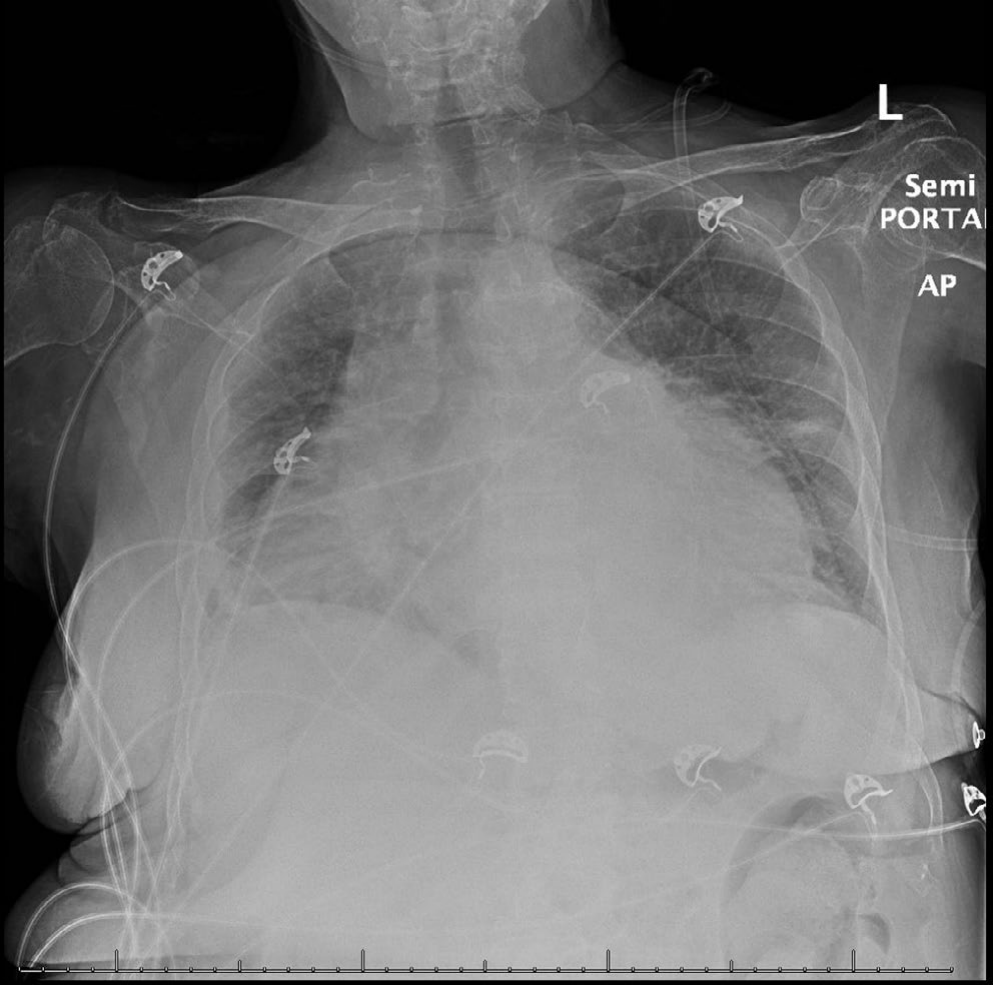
Chest radiograph demonstrating enlarged cardiac silhouette
